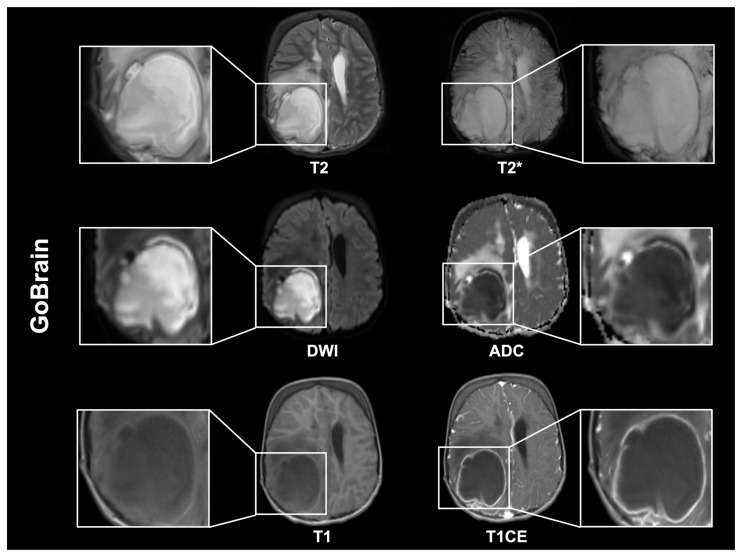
An MRI scan was performed on a 9-year-old male patient using the GOBrain protocol for the management of a brain abscess that required intensive care. Brain abscesses are focal central nervous system (CNS) infections that most commonly result from hematogenous spread, direct extension from adjacent infections (e.g., otitis or sinusitis), or occur after trauma or neurosurgical procedures. The natural progression of the condition is from the initial stage of cerebritis to the formation of a mature capsule. The MRI appearance varies according to the stage of the disease. Early lesions are characterized by poor margination, high signal intensity on T2-weighted images (T2), and the presence of diffusion restriction. In the mature stage, a well-defined capsule is evident, frequently T2w hypointense, with a centrally hyperintense core and marked diffusion restriction, manifesting as bright on DWI with low ADC due to viscous purulent contents. T2*-weighted sequences have been shown to exhibit a characteristic dual-rim sign, thus facilitating the differentiation of abscesses from glioblastomas [20,21]. On non-contrast T1-weighted images, the center is hypointense, whereas post-contrast images typically demonstrate ring enhancement.
